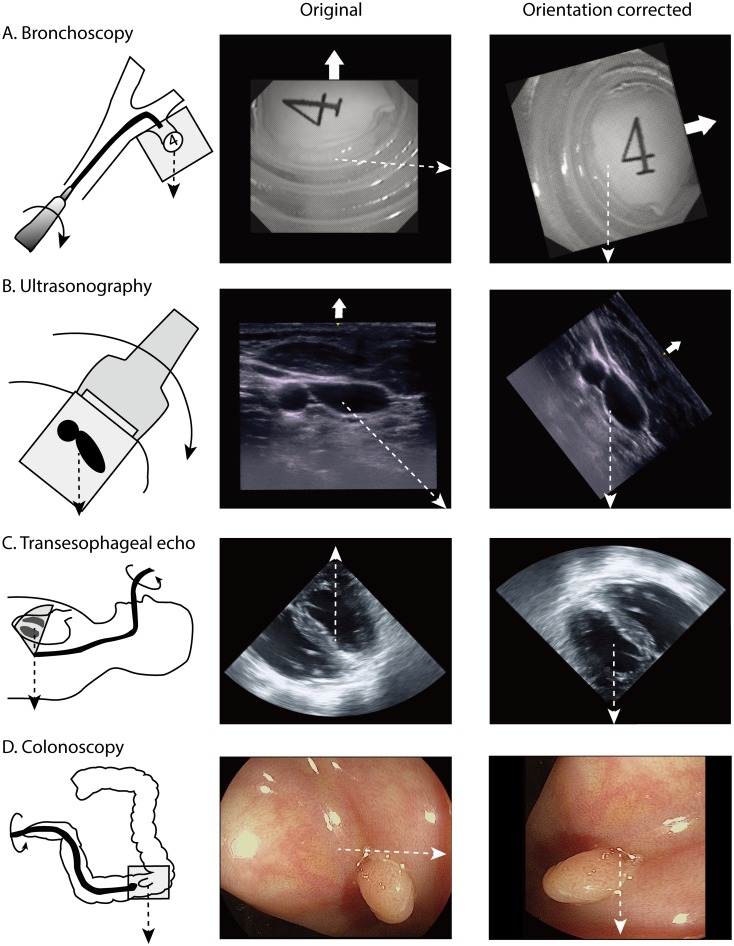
Images before and after orientation correction in various clinical situations. A. With flexible endoscopy, changing the direction requires axial rotation of the endoscope. The gravitational distortion due to the rotation can be corrected by the orientation correction system. The procedure is also easier because the direction of the endoscope tip coincides with the spatial orientation of the endoscopist. This image was captured using the orientation correction system used in this study. B. With ultrasound-guided catheter insertion, the image orientation correction system can help to match the spatial orientation of the image and the operator. This image was captured using the orientation correction system used in this study. C. With conventional transesophageal echocardiography, the direction of gravity is upward. Therefore, air bubbles come down from the top of the image. D. In colonoscopy, the location of the mass can be accurately described even when there is no structural landmark. The upper direction of the raw image is indicated by a thick white arrow and the direction of gravity is indicated by a dashed line.
